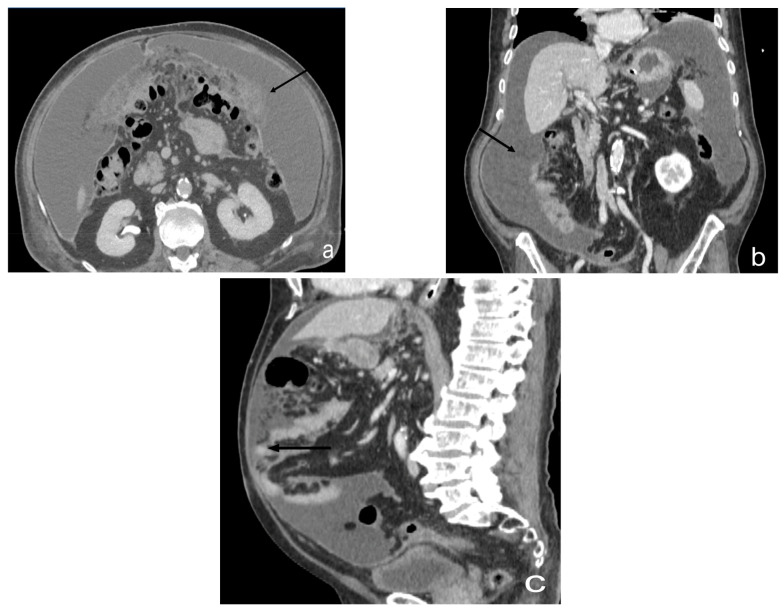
Contrast-enhanced CT scan, axial view (a), coronal view (b), and sagittal view (c). Case of ascitis and omental cake in a male, 76 y. Massive ascitic effusion (black arrow) in the supramesocolic and submesocolic region, with thickening of the omentum and nodular appearance of “omental cake.”
